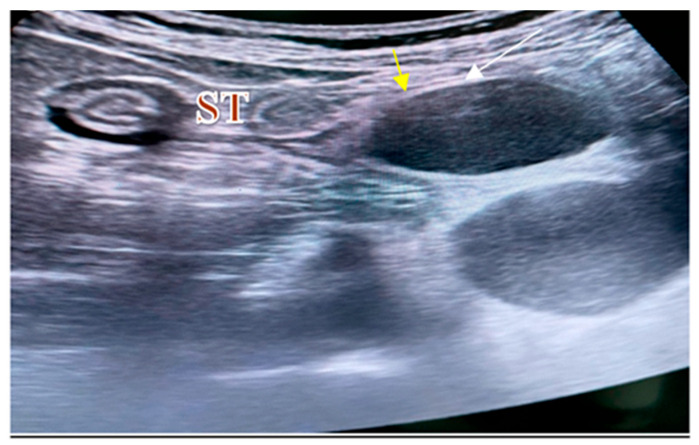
Ultrasound view of a GDC: the inner hyperechoic epithelial lining consists of the alimentary tract mucosa (white arrow) and the exterior hypoechoic layer of smooth muscle (yellow arrow), closely affixed to the gastrointestinal tract by sharing a common wall; ST: stomach. (Personal collection).
